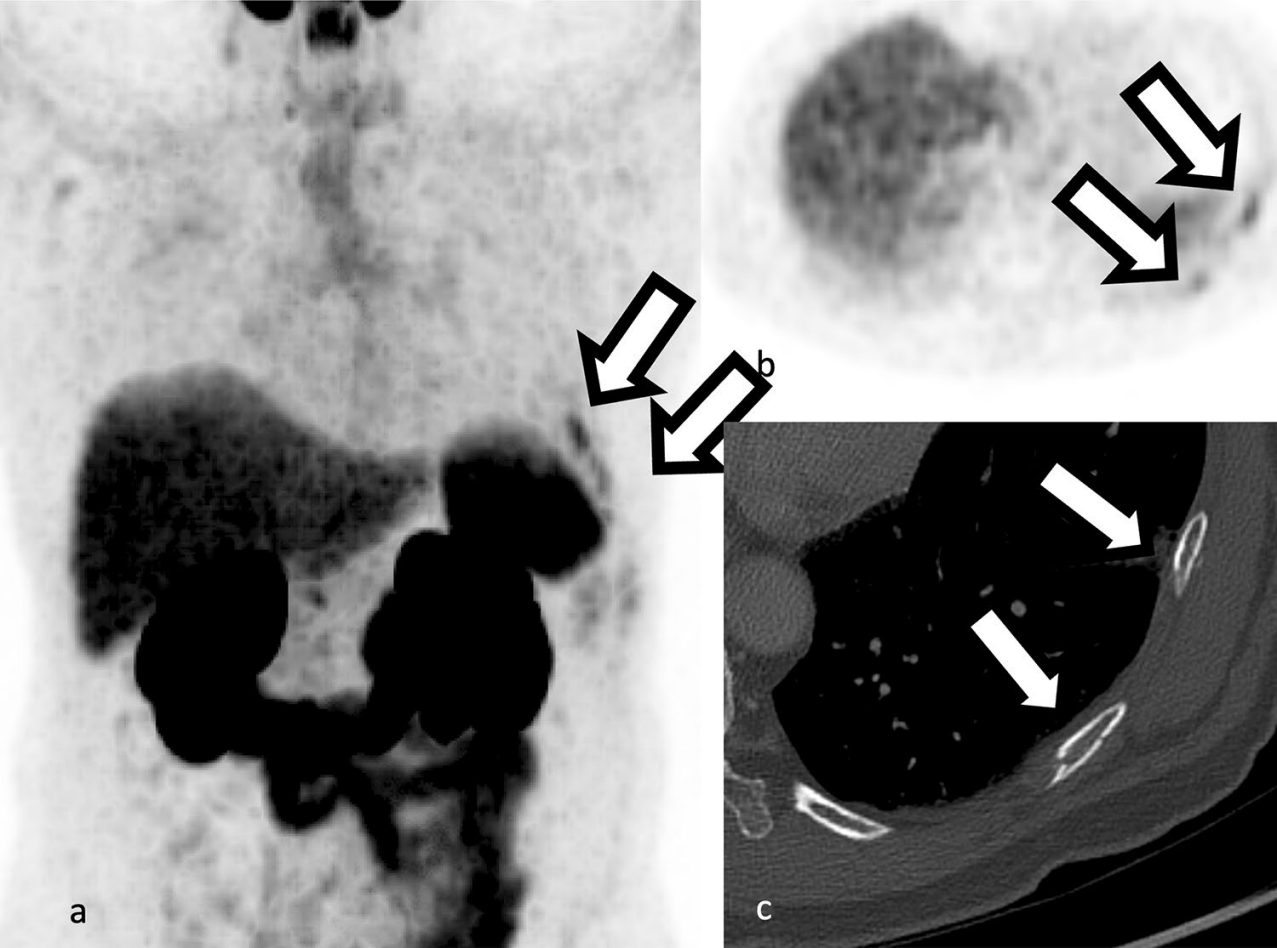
a, b 68Ga-PSMA-11 PET uptake due to fracture, better depicted on corresponding CT (c). Both a and b are scaled to 0–5 SUV.
